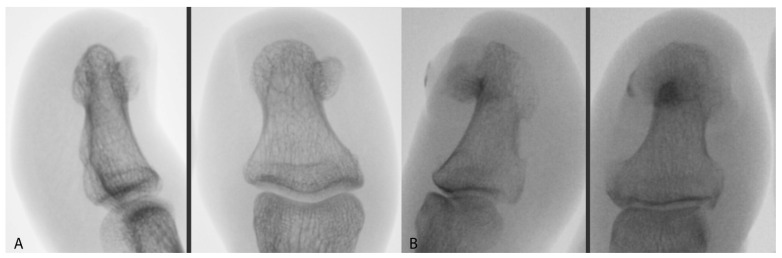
AP and lateral X-ray image of (A) SO and (B) SE.
